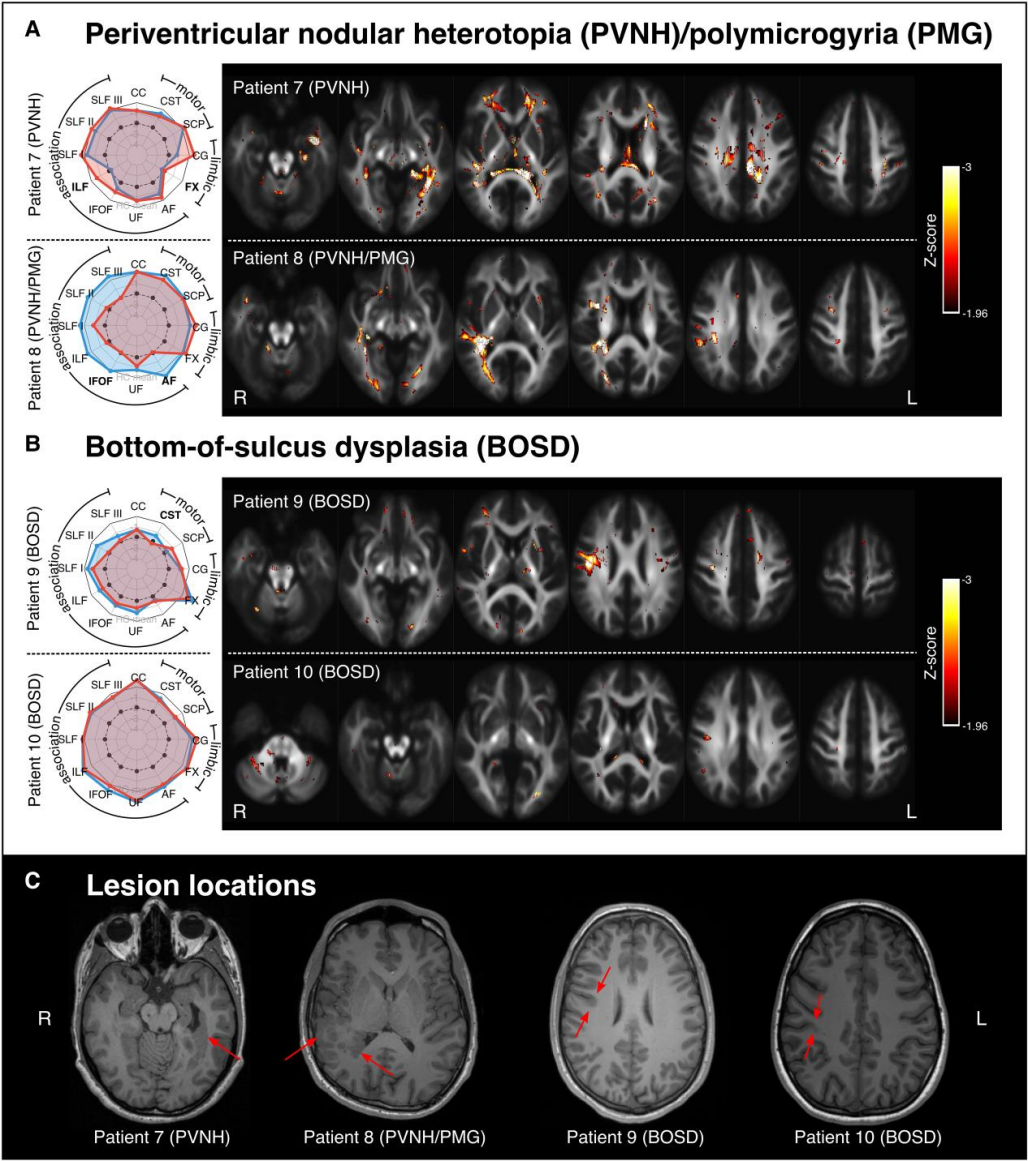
Results in lesional epilepsies. (A) Pane shows individual-level results for two patients with PVNH, one with additional PMG. In both cases, lesions were unilateral, with Patient 7 exhibiting left-sided PVNH, and Patient 8 exhibiting right-sided PVNH and PMG. (B) Panel shows individual-level results for two patients with BOSD: Patient 9 had a small BOSD in the right precentral sulcus; Patient 10 had a BOSD in the right post-central sulcus. See Fig. 2 for spider plot interpretation for Panels A and B. (C) Panel shows the lesion locations for all patients (Patients 7–10) on a single axial slice from a T1-weighted image in each subject’s own space.
